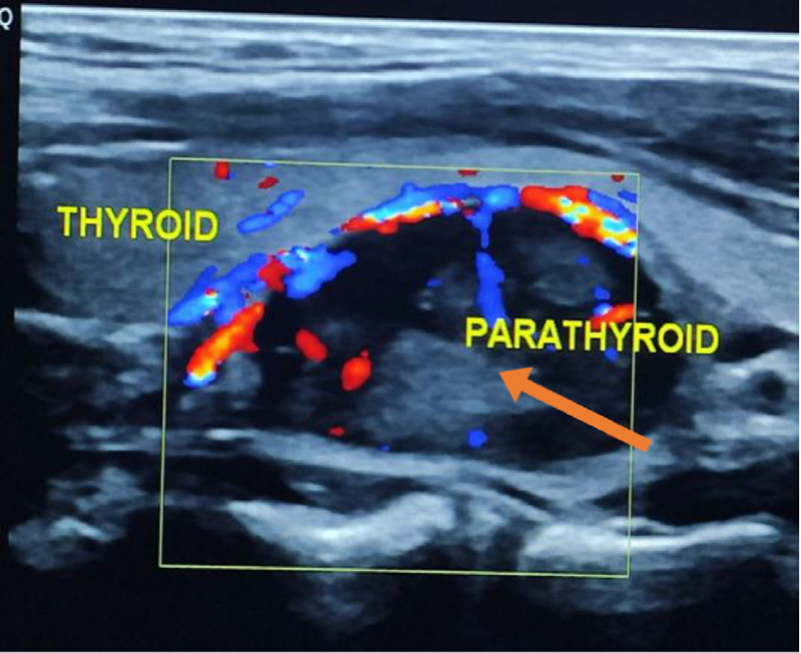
Neck ultrasound images show a hypoechoic, well-circumscribed mass with color-Doppler of monopolar vascular pattern referred to “as the arc or rim sign,” located posteriorly inferiorly to the lower pole of the left thyroid lobe.
